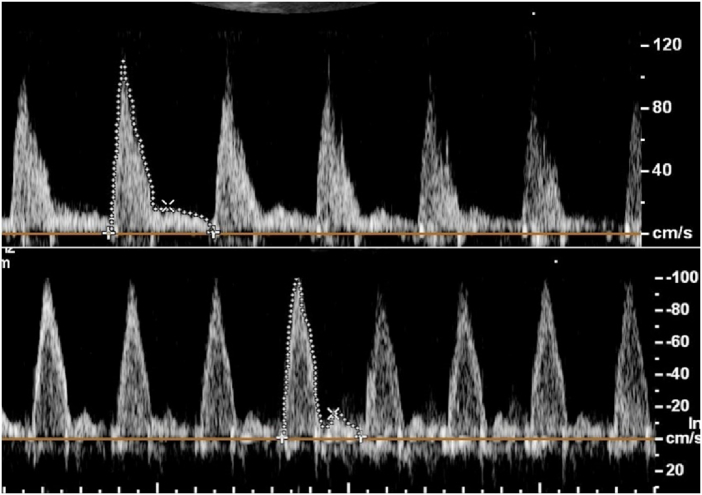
Doppler evaluation of the ductus arteriosus indicated higher peak systolic and diastolic velocities following the administration of betamethasone. In this particular instance, the initial systolic and diastolic velocities were: 98 cm/s and 15.7 cm/s (lower row), which subsequently increased to 108 cm/s and 17.2 cm/s respectively, 40 hr post-betamethasone administration (Upper row).
